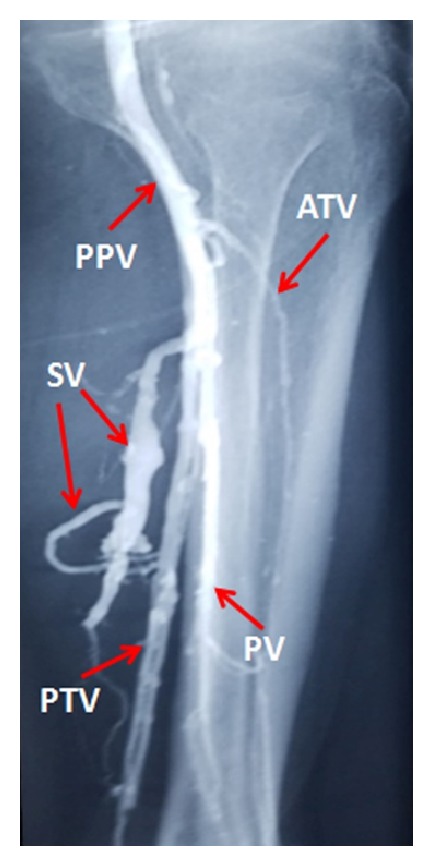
Lateral radiograph of soleal veins by venography. SV, soleal vein; PPV, popliteal vein; ATV, anterior tibial vein; PTV, posterior tibial vein; PV, peroneal vein.
